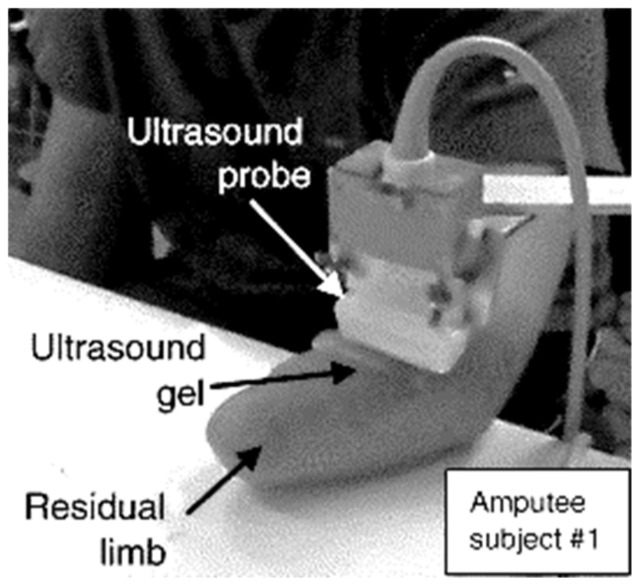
An example of a setup for collecting ultrasound images from the residual forearm of an amputee using a linear ultrasound probe. This figure was adapted from [71] with permission from Elsevier.
